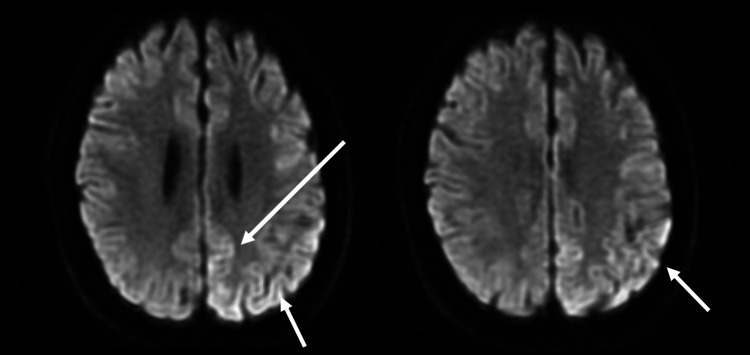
MRI diffusion-weighted images showing a hyperintense signal in the left temporal cortex region
